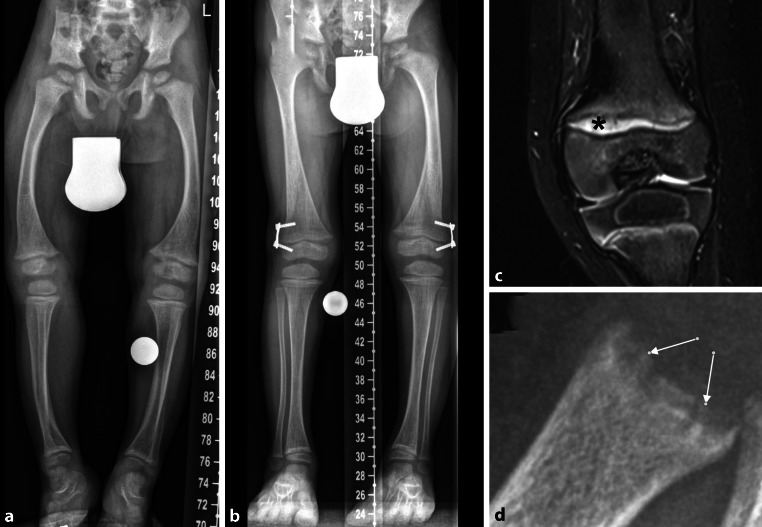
X‑linked hypophosphatemic rickets. a Leg bowing with b significant improvement after bilateral hemiepiphysiodesis. c Physeal widening in MRI (*asterisk* placed on abnormal physis). d Rickets on conventional radiographs (physeal cupping and fraying; *arrows*)
